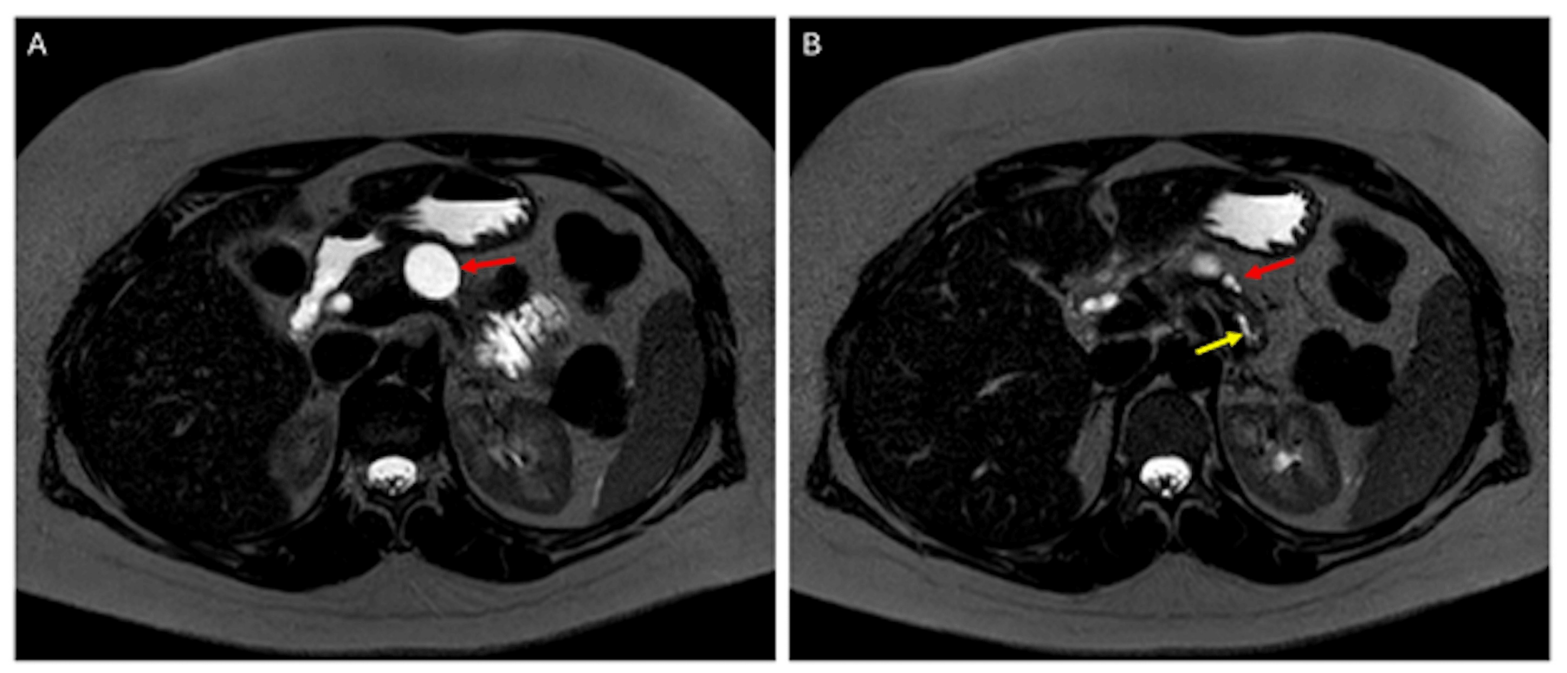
MRI images of remaining pancreatic cysts after resolution of pancreatitis Axial thin MRCP image of the abdomen (A) demonstrates a dominant, T2-hyperintense cyst within the pancreatic body (red arrow). One slice inferior (B), there are two adjacent smaller pancreatic cysts (red arrow) and dilation of the main pancreatic duct within the partially atrophic pancreatic tail (yellow arrow). These findings are consistent with local complications from acute pancreatitis and showcase the development of chronic pancreatitis. MRI: magnetic resonance imaging; MRCP: magnetic resonance cholangiopancreatography
